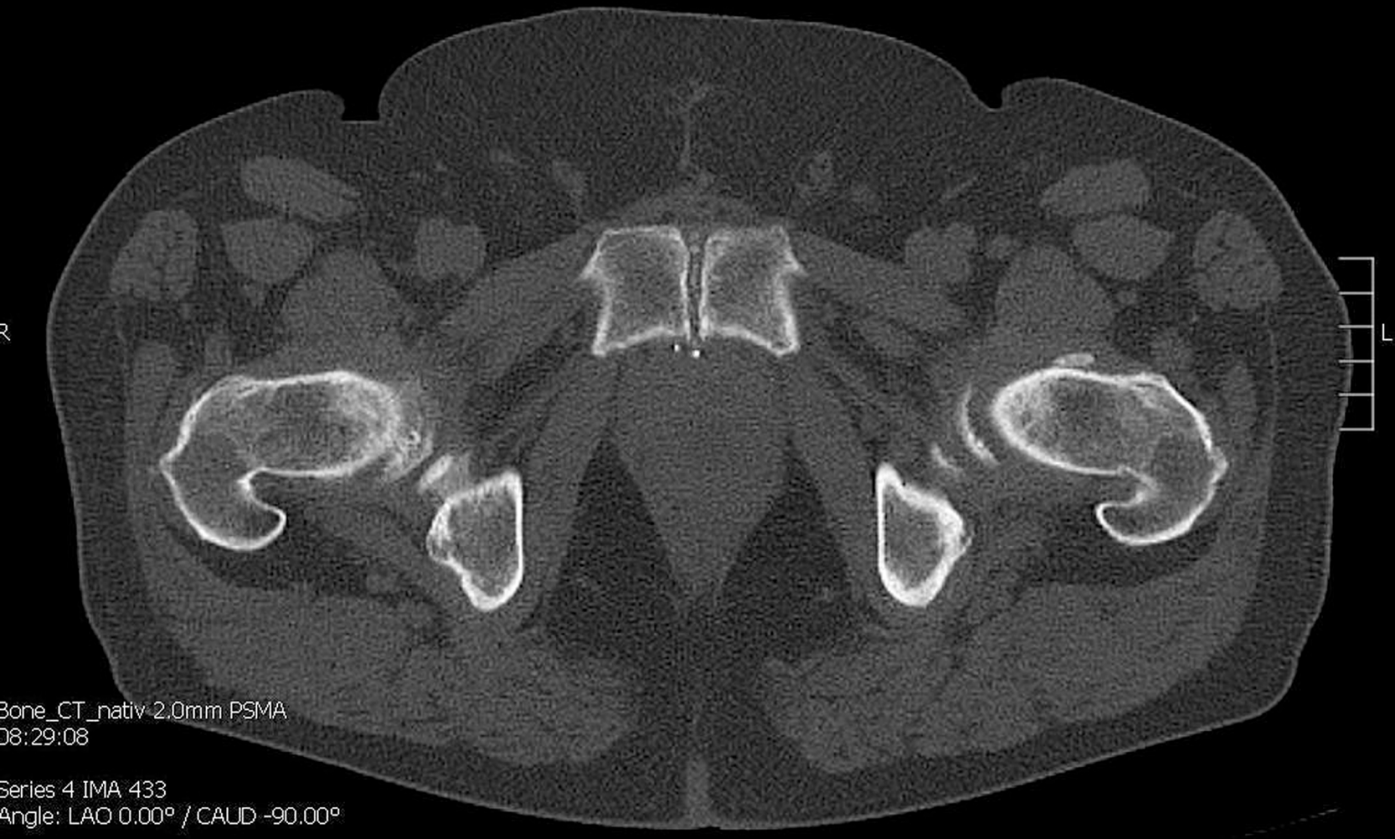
CT shows no bone metastasis in the symphyseal os pubis on the left.
